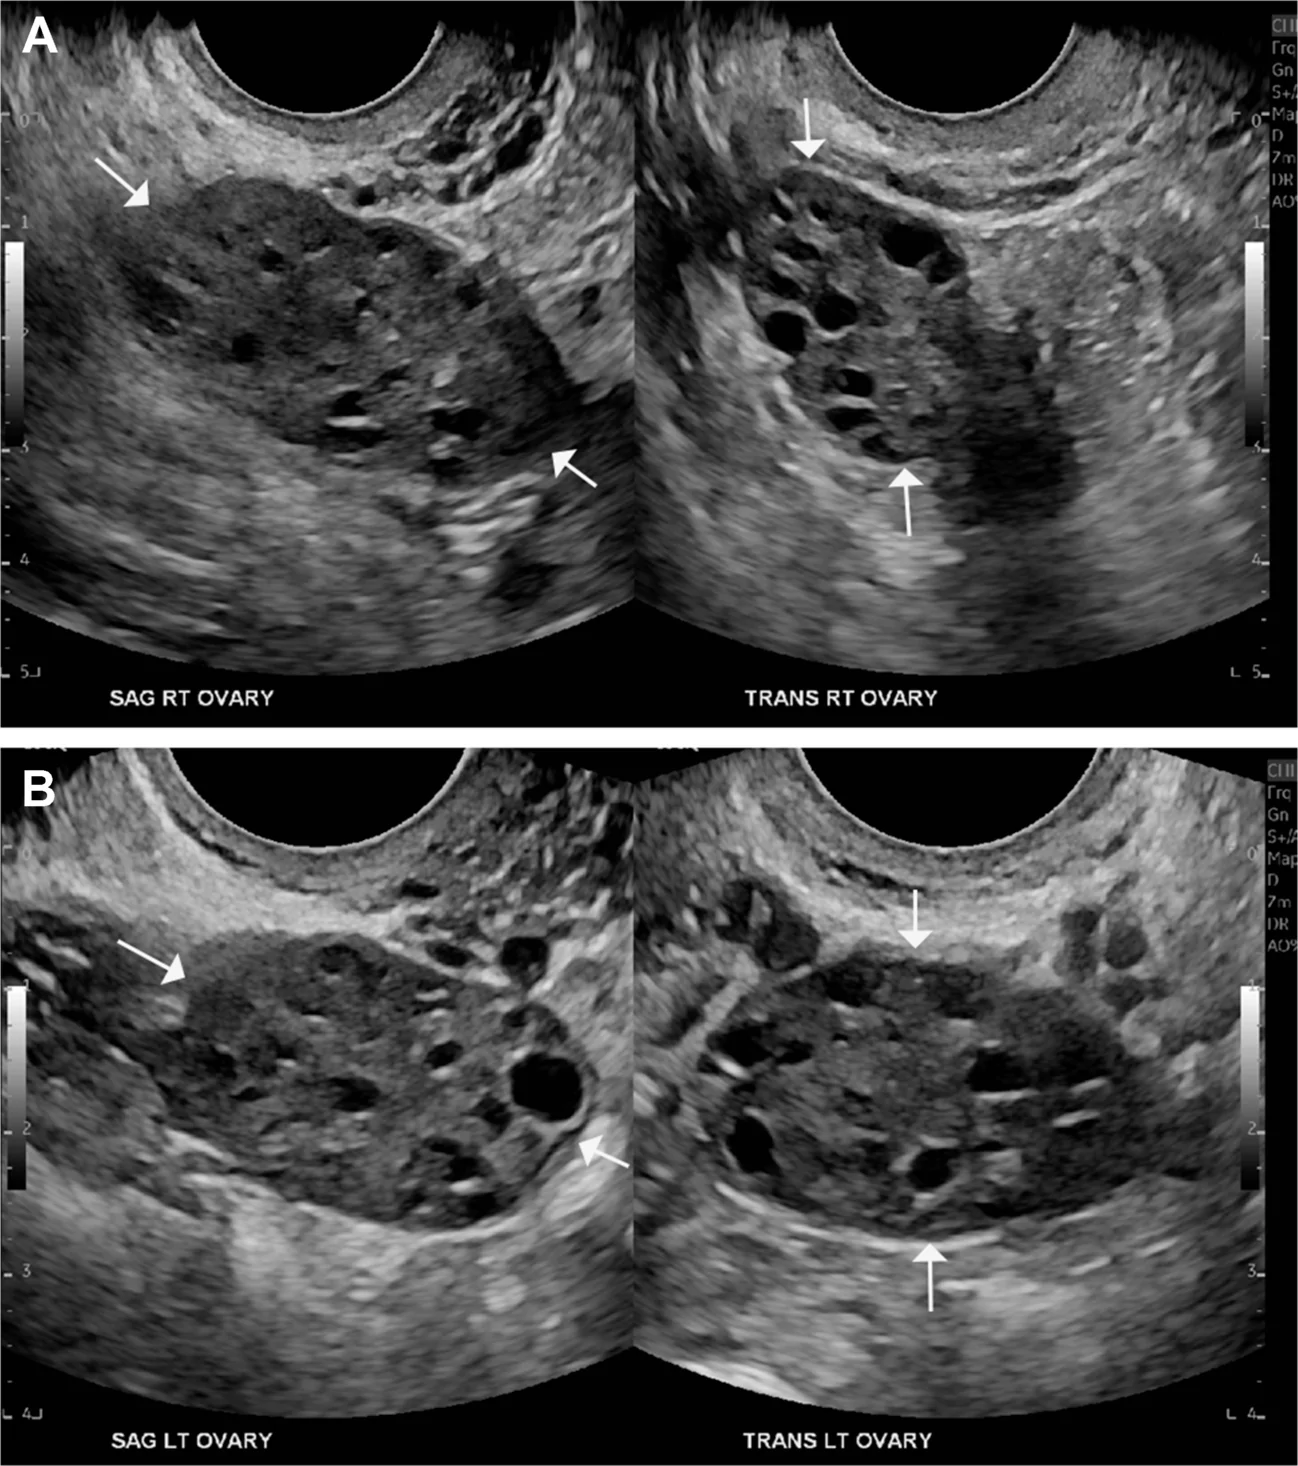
A 20-year-old female with polycystic ovarian syndrome (PCOS), presenting with secondary amenorrhea, obesity, and hirsutism. Transvaginal ultrasound images show abnormally enlarged (a) right and (b) left ovaries (*arrowheads*) with numerous small follicles typical of PCOS morphology. Right ovarian volume is 18 mL; left ovarian volume is 20 mL
